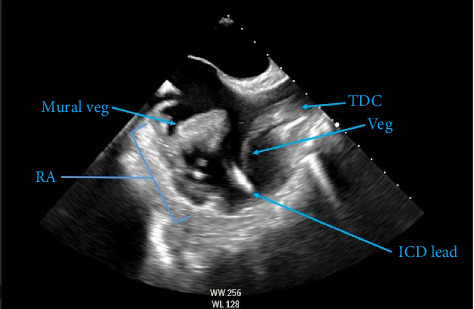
Transesophageal echocardiogram showing the right atrium (RA) with vegetations (veg) attached to the right atrial wall (mural veg) as well as intimately associated with a tunneled dialysis catheter (TDC) and implantable cardioverter–defibrillator (ICD) lead.
